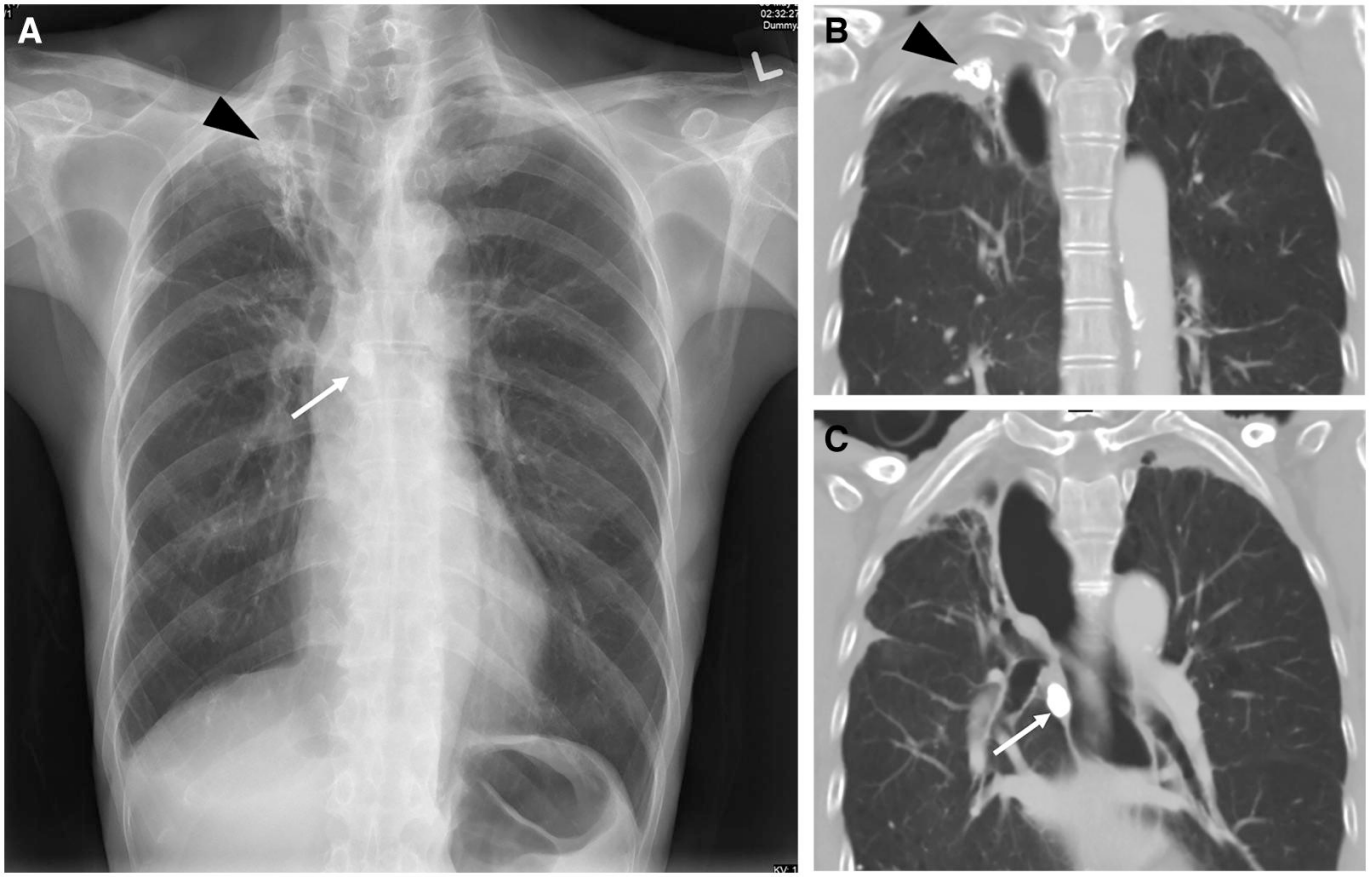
Previous pulmonary TB with Ranke complex in an 84-year-old man. (A) Frontal chest radiograph shows right upper lobe fibrosis (black arrowhead) with right hilar calcification (white arrow). (B and C) Coronal CT images better show the right upper lobe fibrocalcific lesion (black arrowhead) as well as right tracheobronchial calcified lymph node (white arrow).
